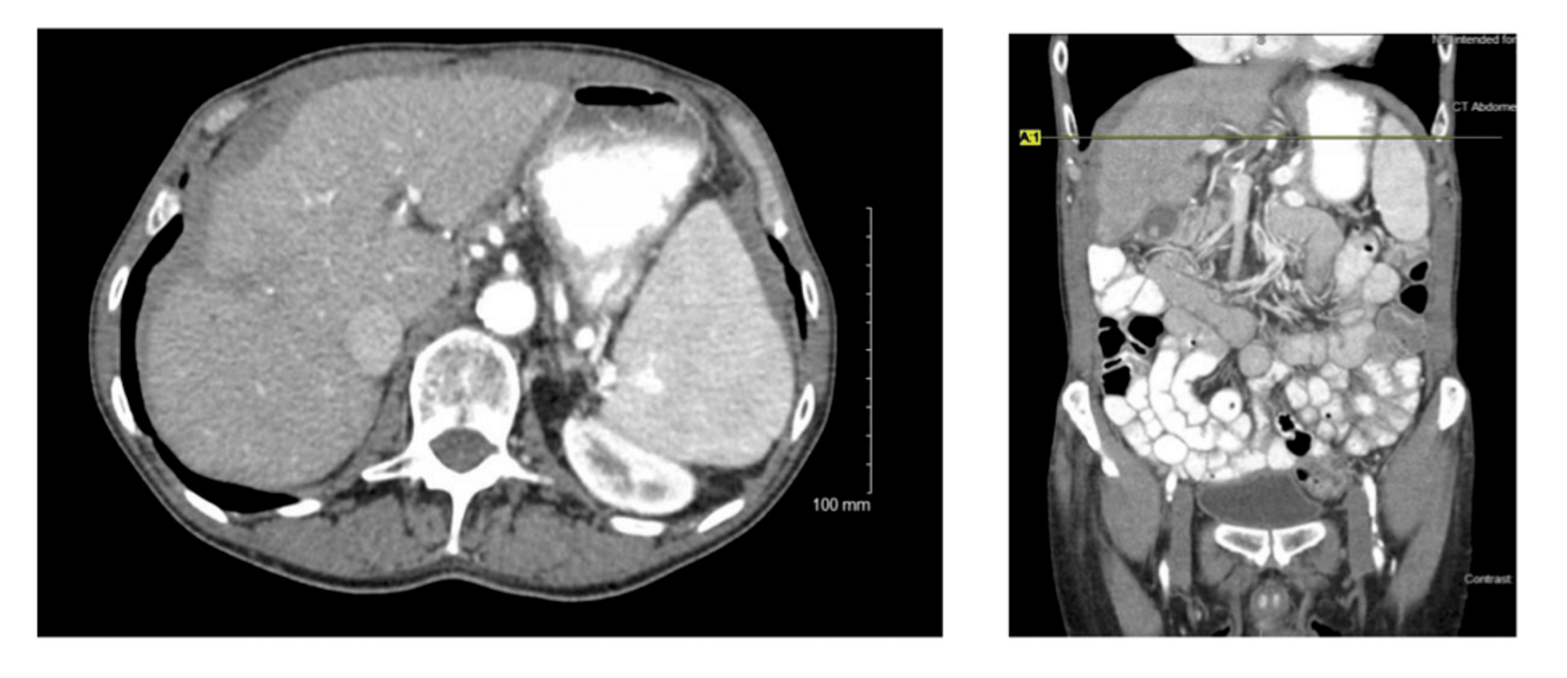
Preoperative CT images Fibrotic-appearing liver and perihepatic hypoattenuating substance interpreted as ascites.
